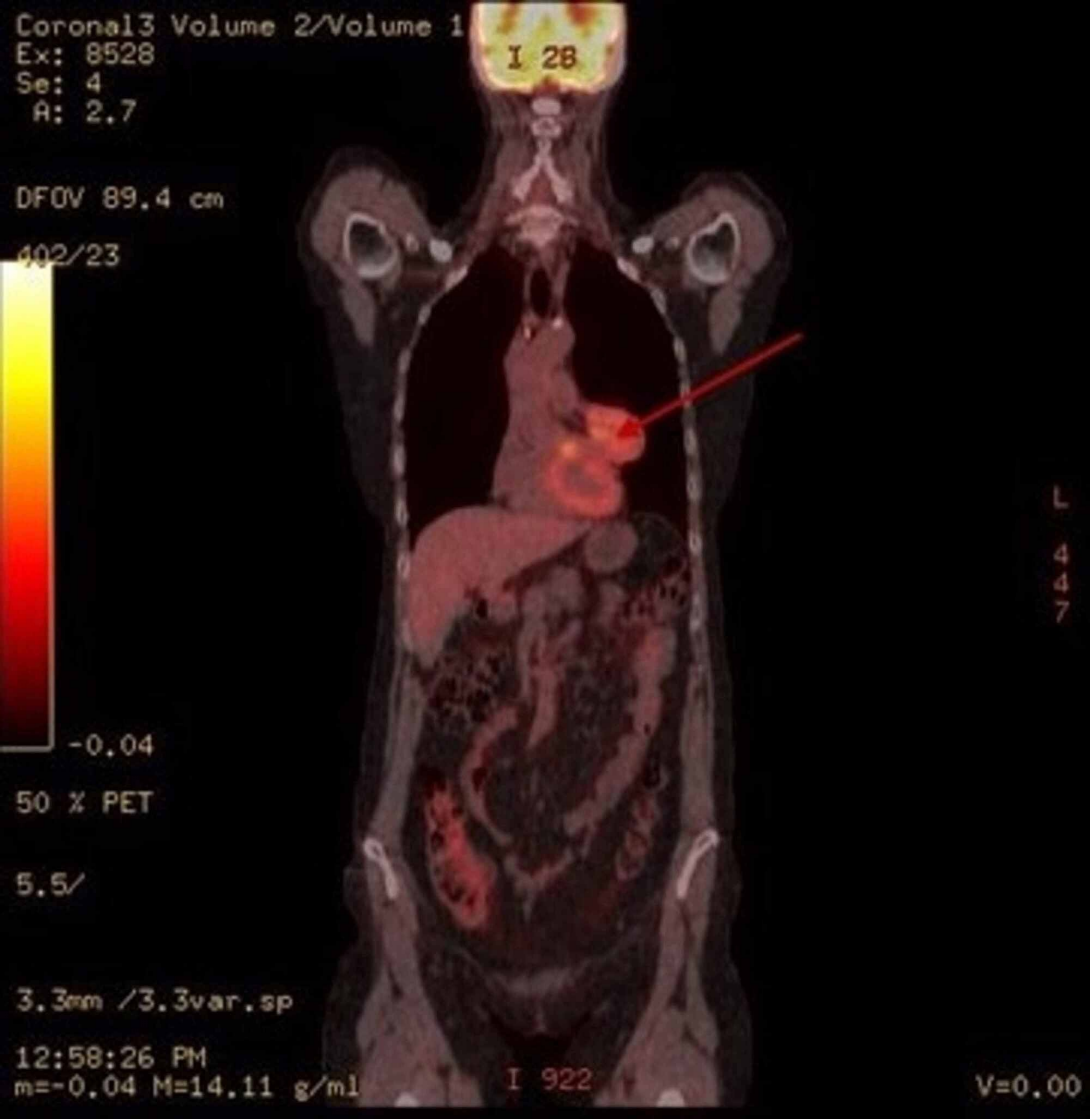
PET scan indicated the anterior mediastinal mass had an increased F18 FDG uptake with SUV of 7.2. SUV: standardized uptake value; PET: positron emission tomography.
